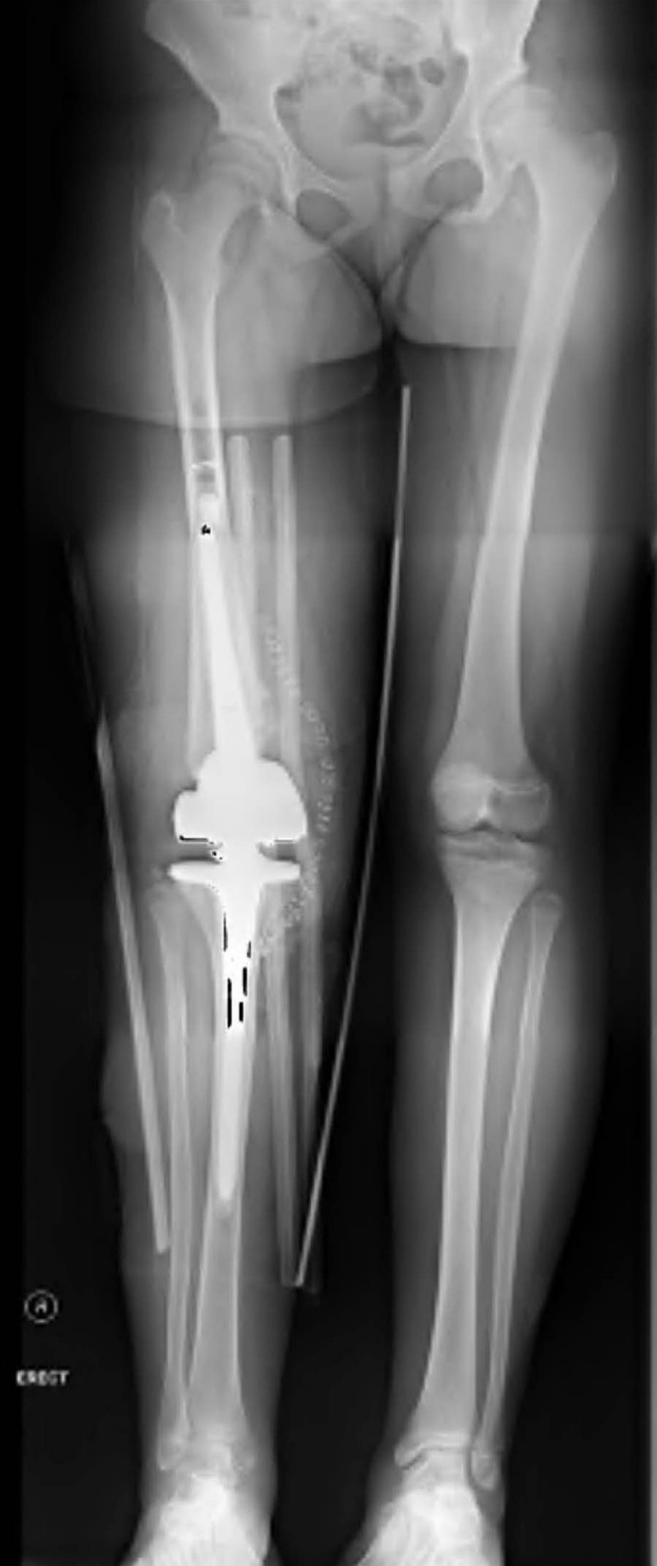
Full-length anteroposterior radiograph of both lower limbs showing restored alignment of the right knee.
